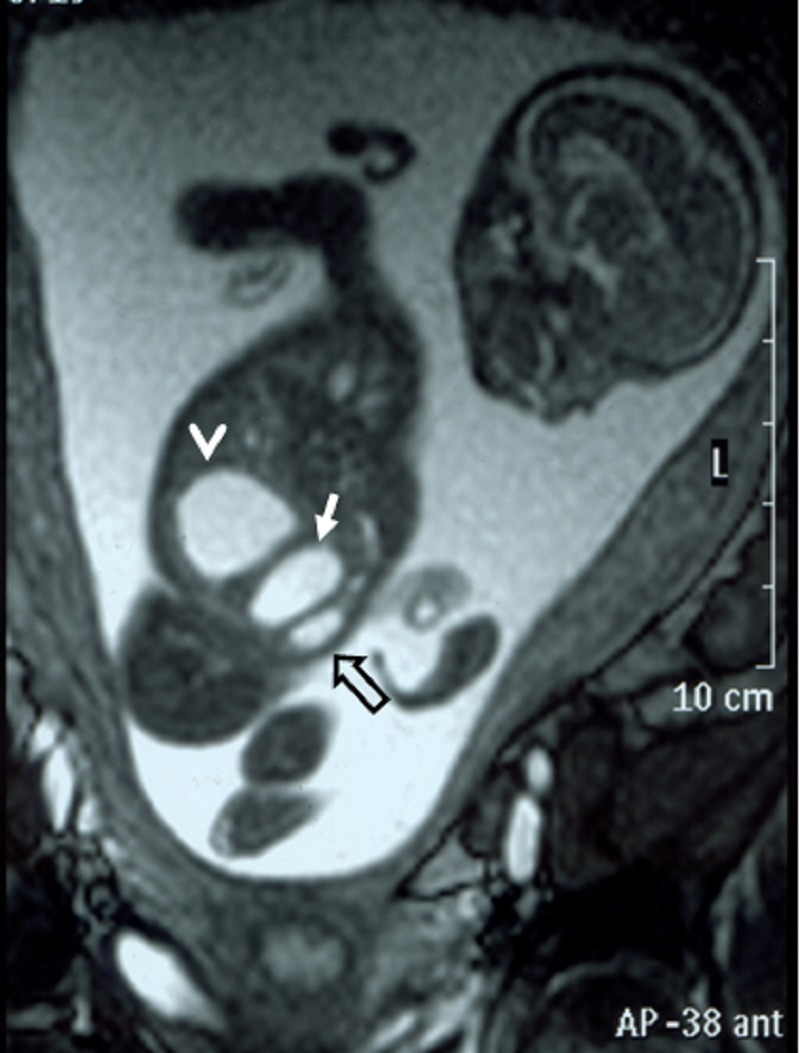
Figure 5: Fetal MRI coronal plane.
